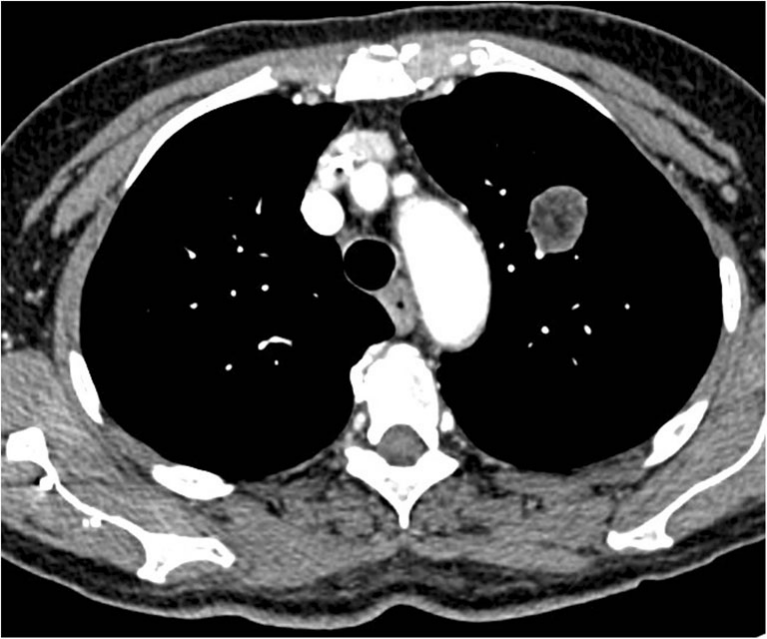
Axial chest CT scan, mediastinal window setting, in a 69-year-old woman with a previous history of gallbladder carcinoma. Because of increase in tumour markers, an 18F-FDG-PET-CT was ordered, showing a well delineated round nodule with absence of 18F-FDG-uptake. The internal aspect of the nodule shows small hypodense foci with negative Houndsfield units on measurement. The typical morphology, absence of uptake on 18F-FDG-PET and absence of evolution are consistent with a benign hamartoma
